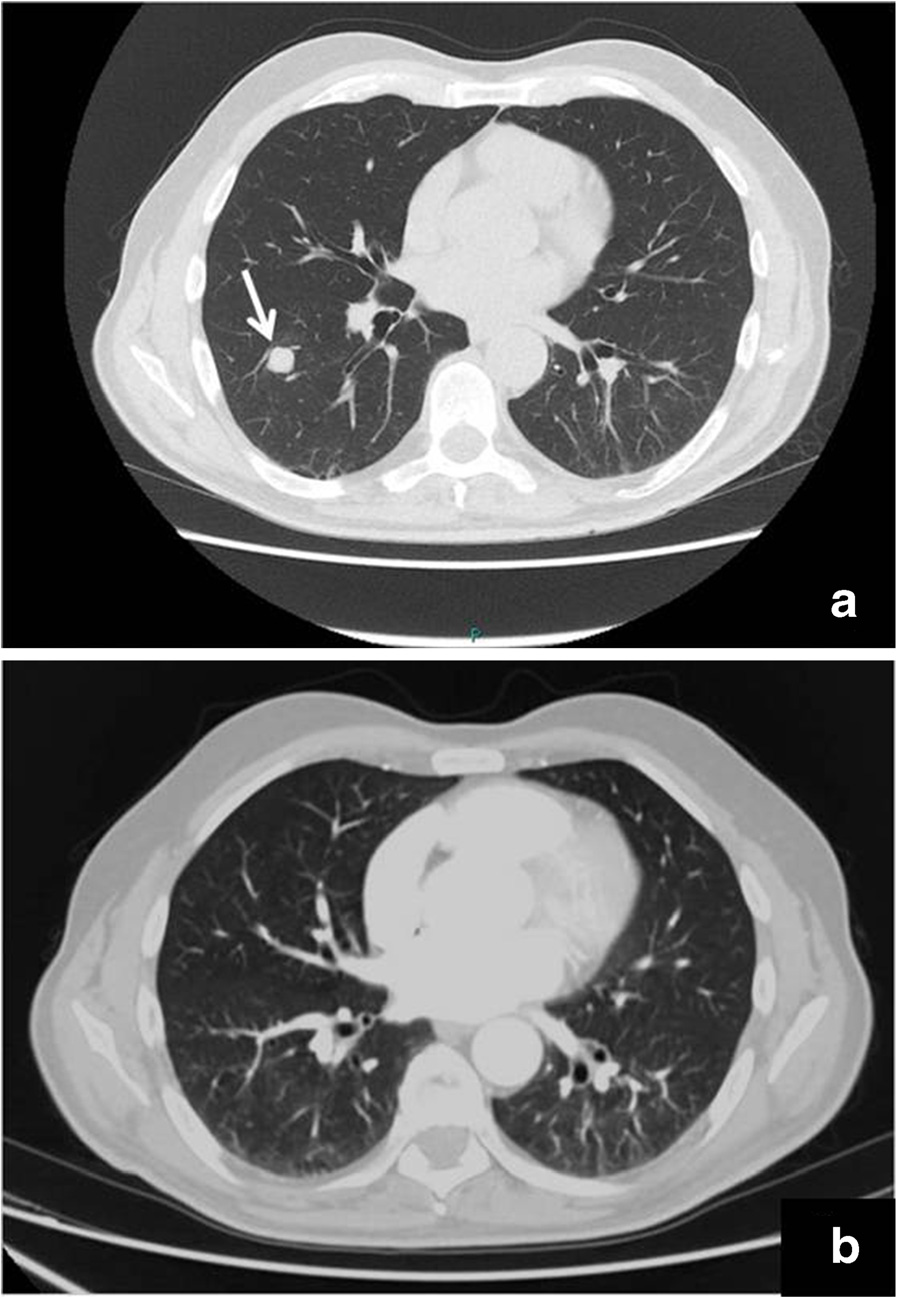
Total-body computed tomography scans with contrast enhancement. Total-body computed tomography scans show: (a) a nodular mass (a ‘cannonball’ - 13x14 mm) in the lower lobe of the right lung (white arrow); (b) at eight-month follow-up, the scan shows the disappearance of the pulmonary lesion.
